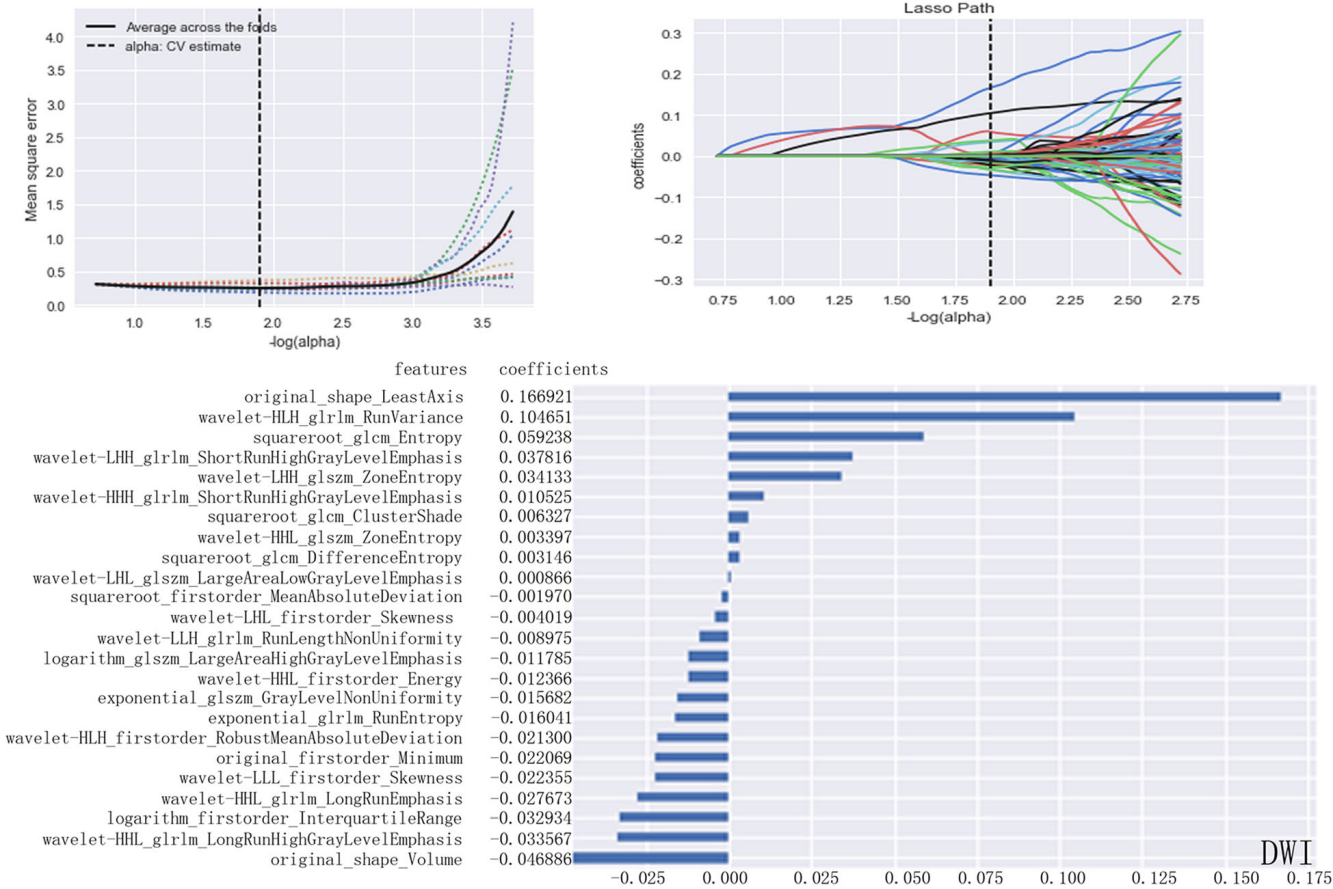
LASSO model on DWI images. The optimal value of the lasso tuning parameter (alpha = 1.903) is found. And 24 features which are correspond to the optimal alpha value are extracted following coefficients on DWI images
